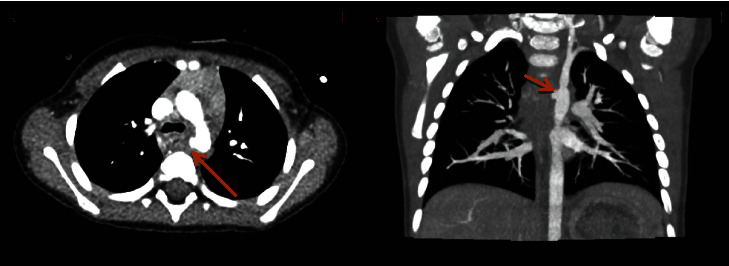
Presenting with hematemesis 7 days after initial EGD, CT angiogram axial (left) and coronal (right) views revealing pseudoaneurysm along the posteromedial wall near the distal aortic arch/proximal descending thoracic aorta just caudal to the takeoff of the left subclavian artery with no evidence of active bleeding.
